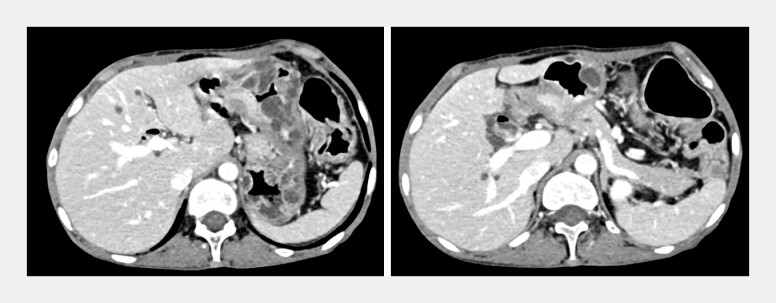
Contrast-enhanced CT reveals localized, relatively prominent, uneven edema and thickening of the gastric wall and duodenal wall, with mucosal enhancement observed during the contrast phase. CT, computed tomography.
